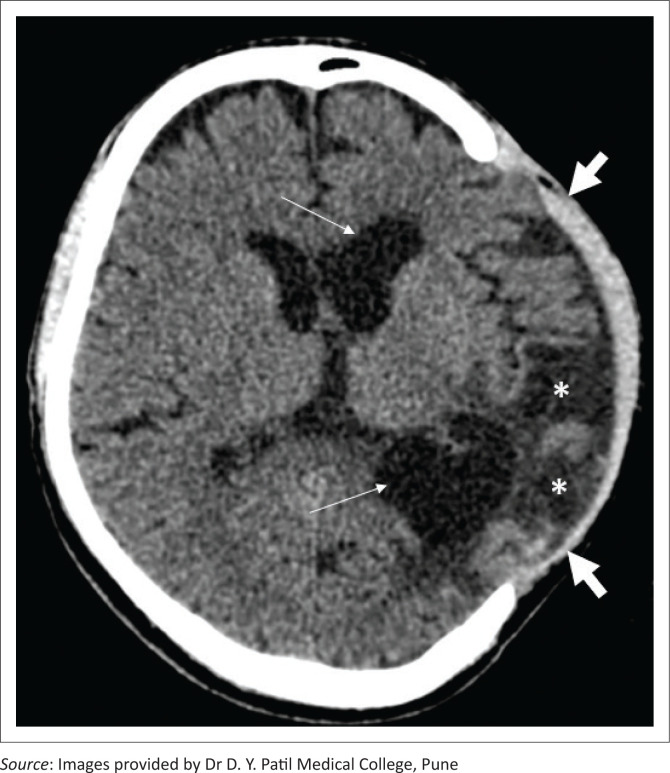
Axial soft tissue window CT image in a patient with a left fronto-parietal decompressive craniectomy indicates herniation of the brain parenchyma through the craniectomy defect (thick white arrows). Extensive encephalomalacia (asterisks) in the left fronto-parietal region along with ex vacuo dilatation of the left lateral ventricle is also seen (white arrows).
